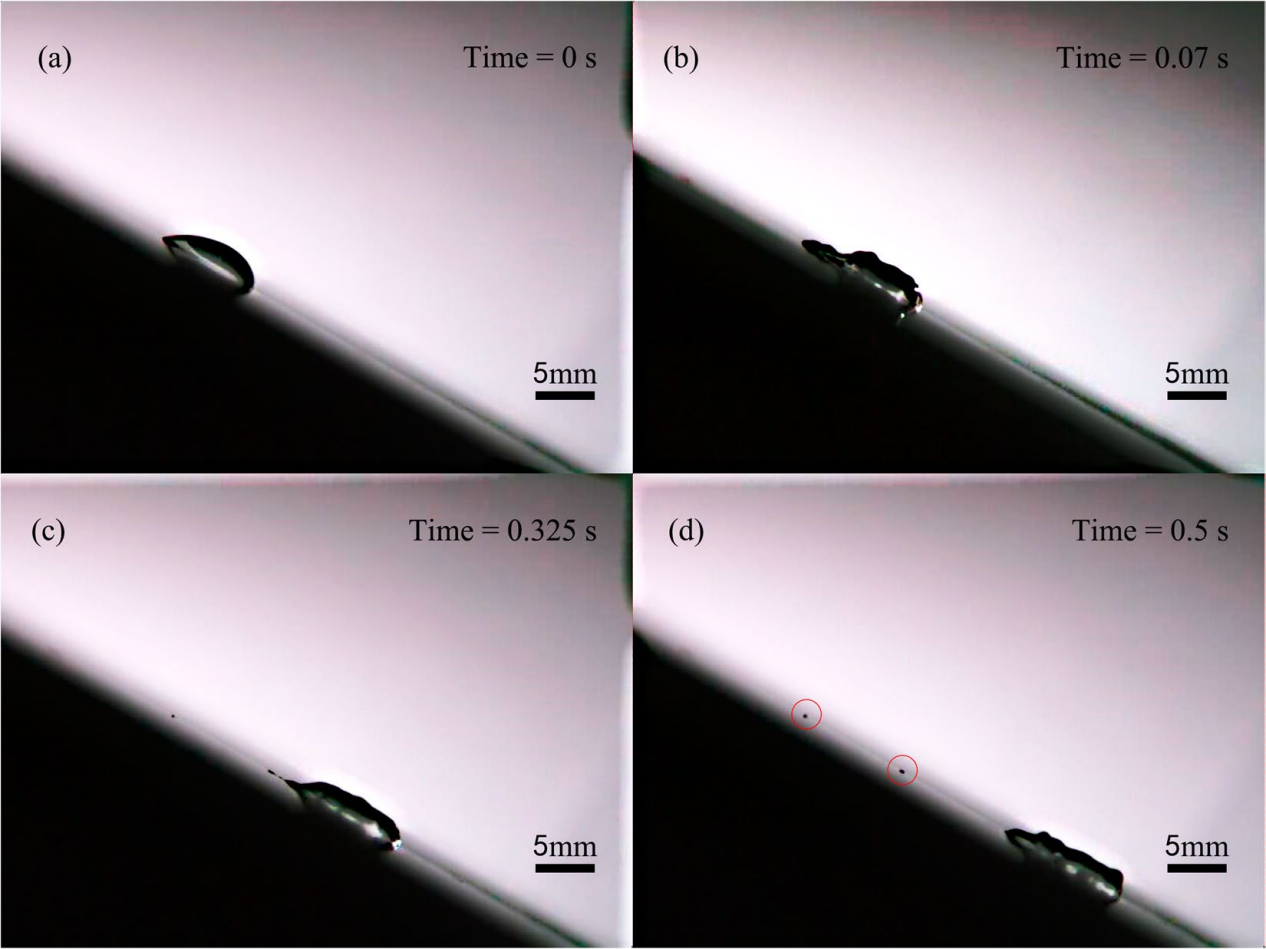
Experimental observation for a 30 μl droplet placed on a 1 mm thick inclined glass plate at an inclination angle of 30° at a wave amplitude of 17.95 nm (measured by laser-Doppler vibrometry) produced by a 1 MHz single phase transducer (SPT) at an input AC voltage 95 V_pp_. The observations are snapshots from video sequences recorded with a high-speed camera. The waves are propagating from left to right. (a) The initial state was at 0 s, (b) the droplet first becomes in a longitudinal expansion at the bottom, (c) above a critical speed, the trailing end breaks up to a pearl, (d) two pearl drops are pinned on the glass plate.
